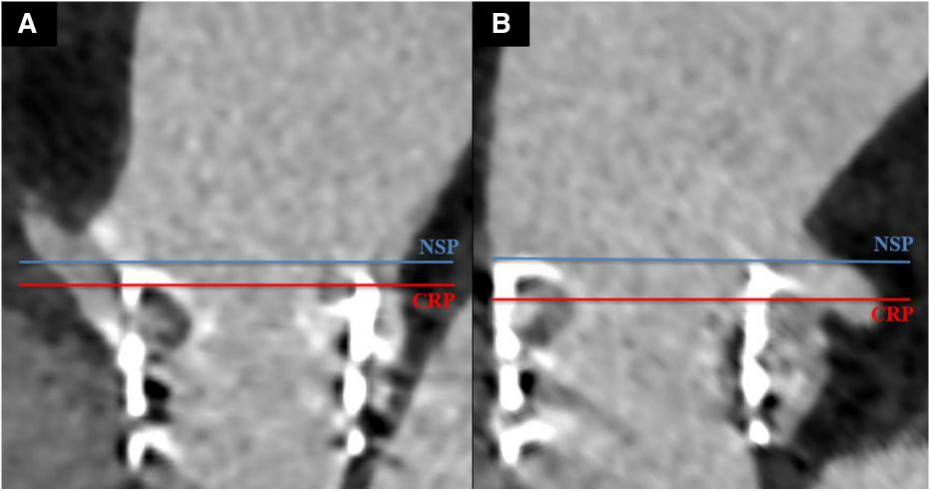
At computed tomography, relationship between coronary risk plane (CRP) and neo skirt plane (NSP) for right coronary artery (*A*) and left coronary artery (*B*).
